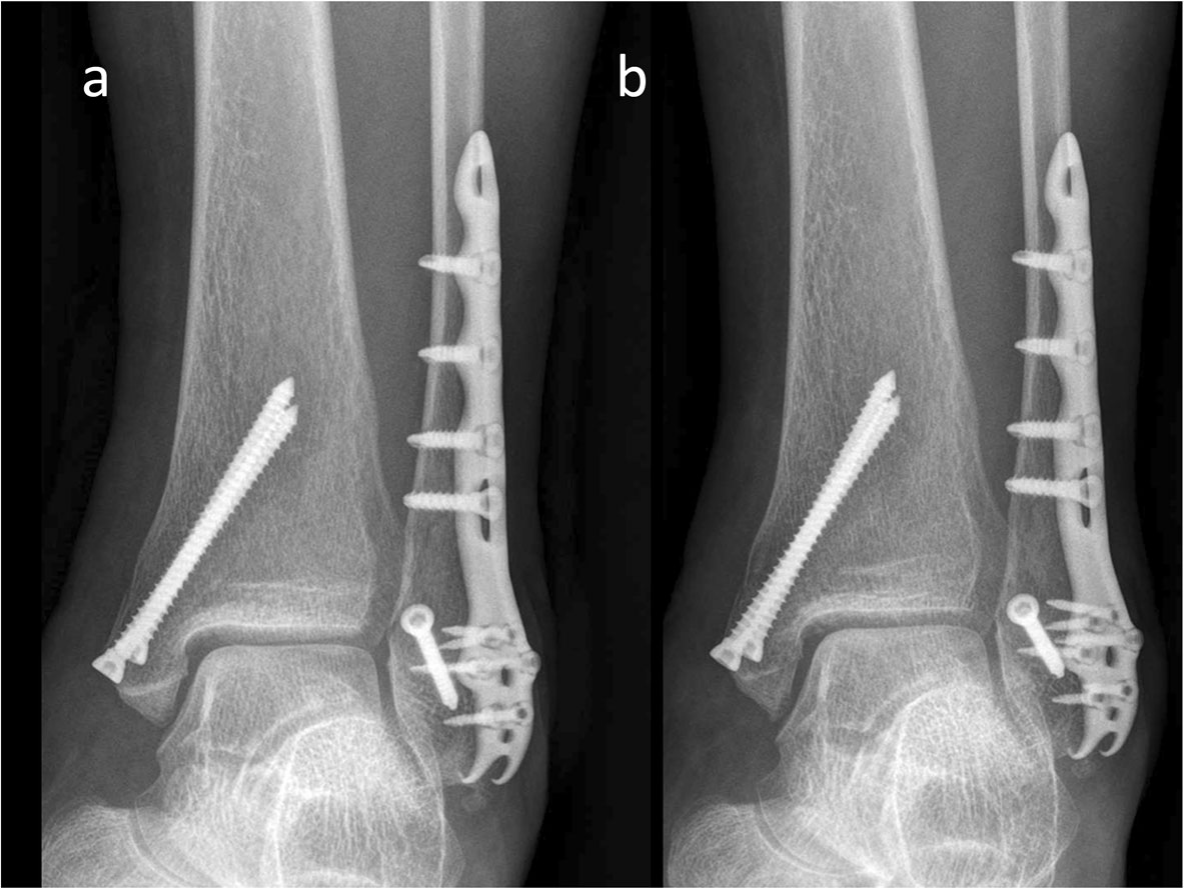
A 64-year-old woman treated using an interfragmentary screw and an Aplus PLP. a The fracture was well-reduced (immediately postoperative radiograph). b It was united without shortening, further displacement, or changes in OA grade
